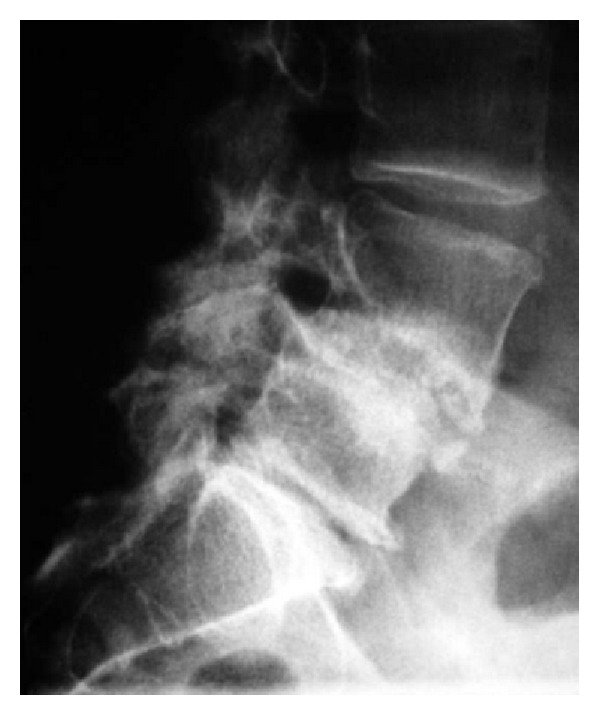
Lateral radiograph shows destruction of L4-L5 vertebral bodies producing multiple bony fragments (Case 2).
